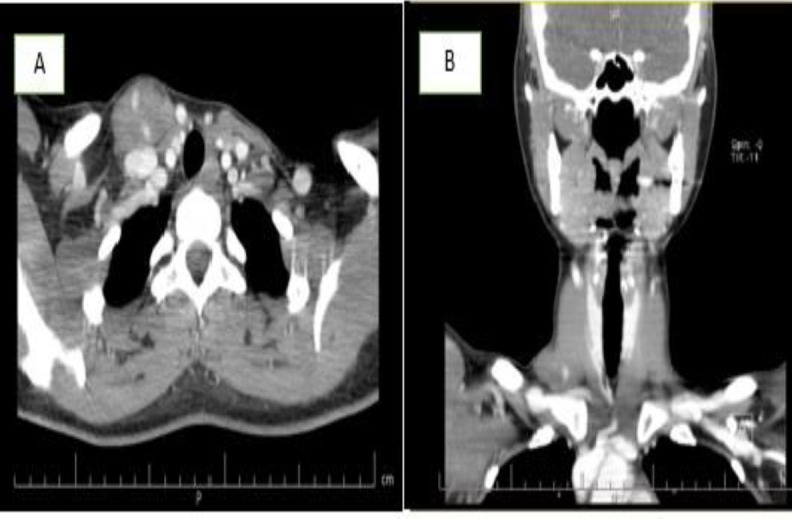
Axial (A) and coronal (B) CT images show heterogeneous enhancing solid mass measured 40×25×20 mm infiltrating the most distal part of the right sternocleidomastoid muscle in the base of the neck containing mottled hyperemic foci
